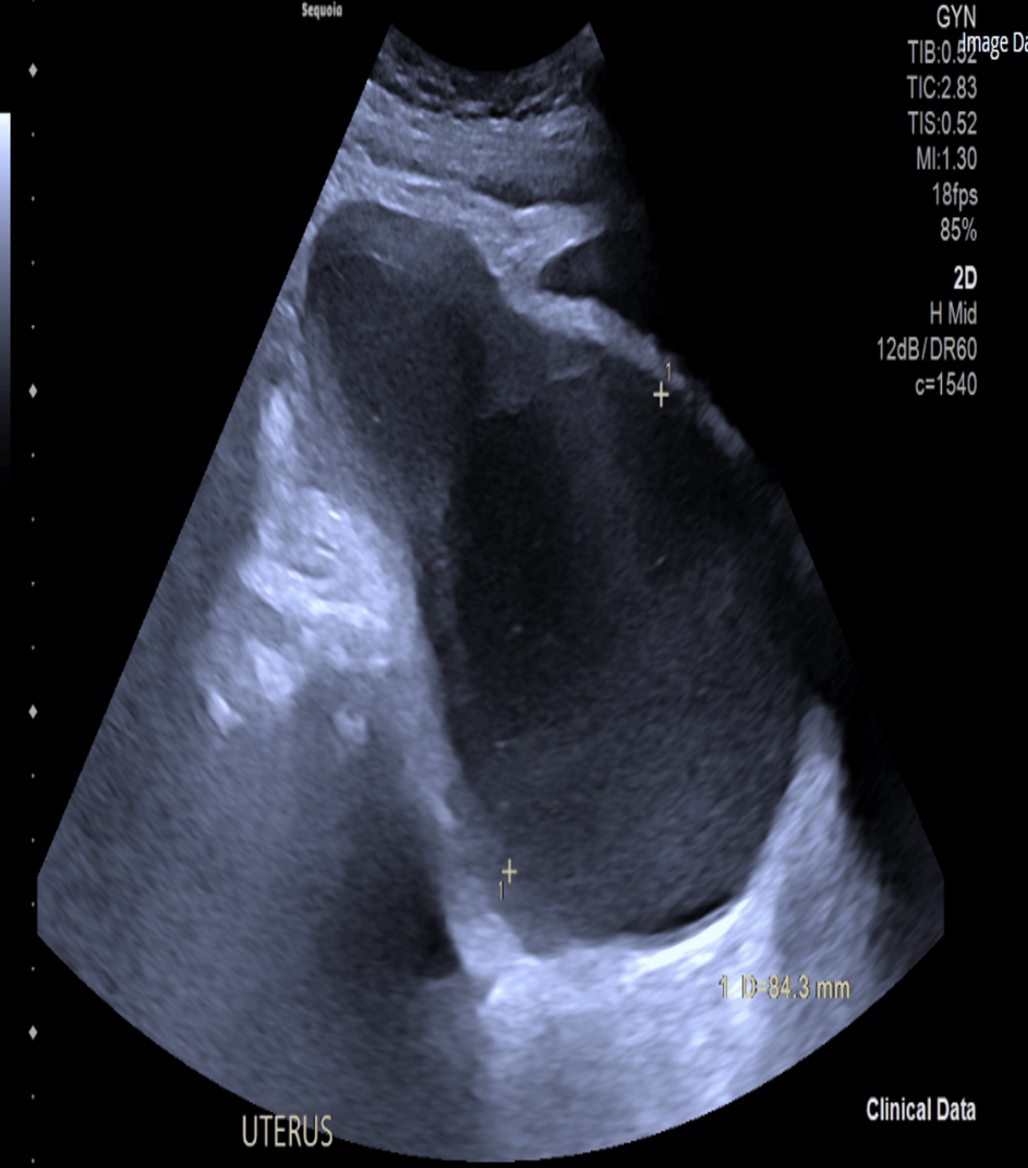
Repeat pelvic ultrasound on third presentation showing hematometra. Again, the cavity is distended by 17.5 mm and contains homogeneous matter. The cervix contains a homogeneous collection measuring 31 × 29 × 25 mm, with no vascularity seen. The uterus measured 8.4 × 7.2 cm  in size.
